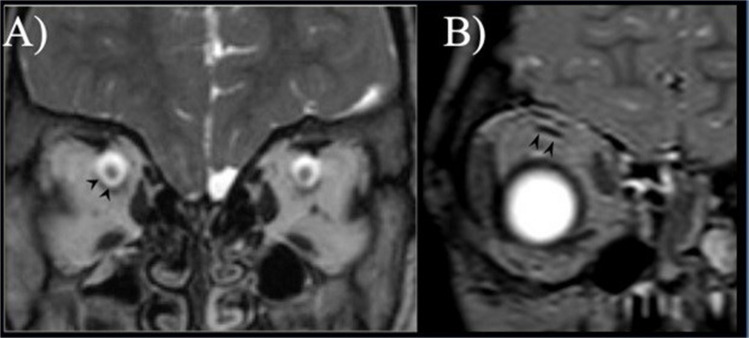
A Coronal T2-weighted image showing the typical target-like appearance of the optic nerve-meningeal sheet complex with the dark optic nerve in the center surrounded by hyperintense perineural cerebrospinal fluid externally lined by the thin dark dural meningeal layer (arrowheads). A diameter greater than 5–6 mm (according to age below or above 1 year) suggests enlargement of the cerebrospinal fluid space due to intracranial hypertension. On the contrary, an optic nerve diameter smaller than 3 mm suggests optic nerve atrophy or hypoplasia. B Coronal T2-weighted image of the right orbit showing a thin superior rectus muscle consistent with severe hypotrophy
